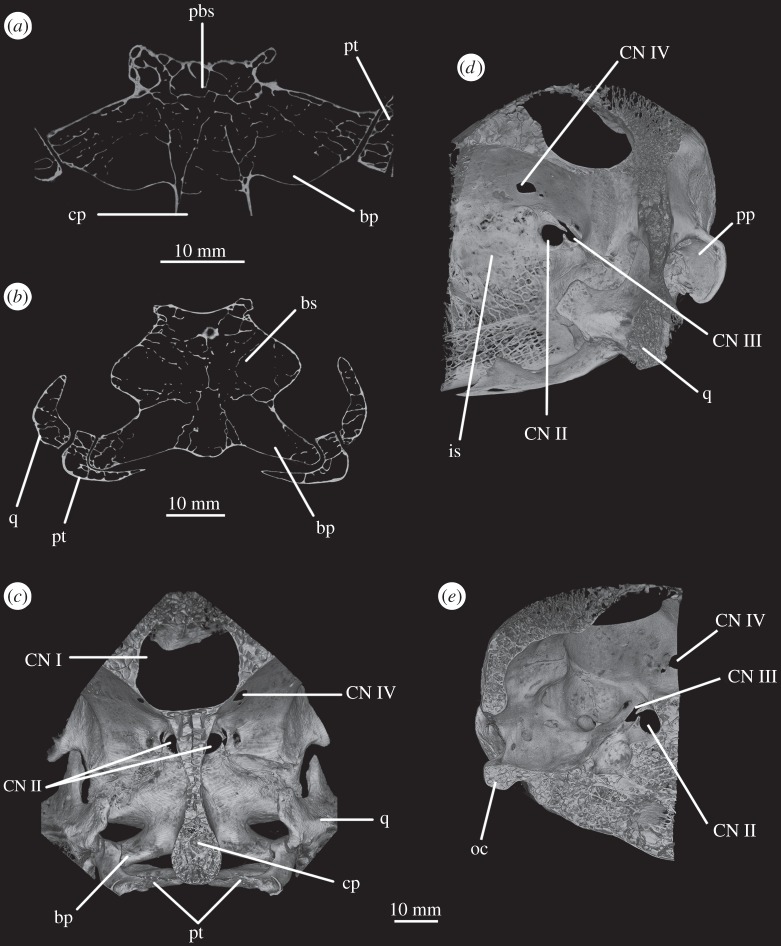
Braincase of *Struthio camelus* (ZMB 2000 2769): (*a*) transverse and (*b*) coronal CT cut of the anterior part of the braincase showing morphology of basipterygoid processes; CT reconstructions of braincase in (*c*) anterior (with posterior part of braincase removed for clarity), (*d*) left anterolateral and (*e*) left medial views. For abbreviations, see table 1.
